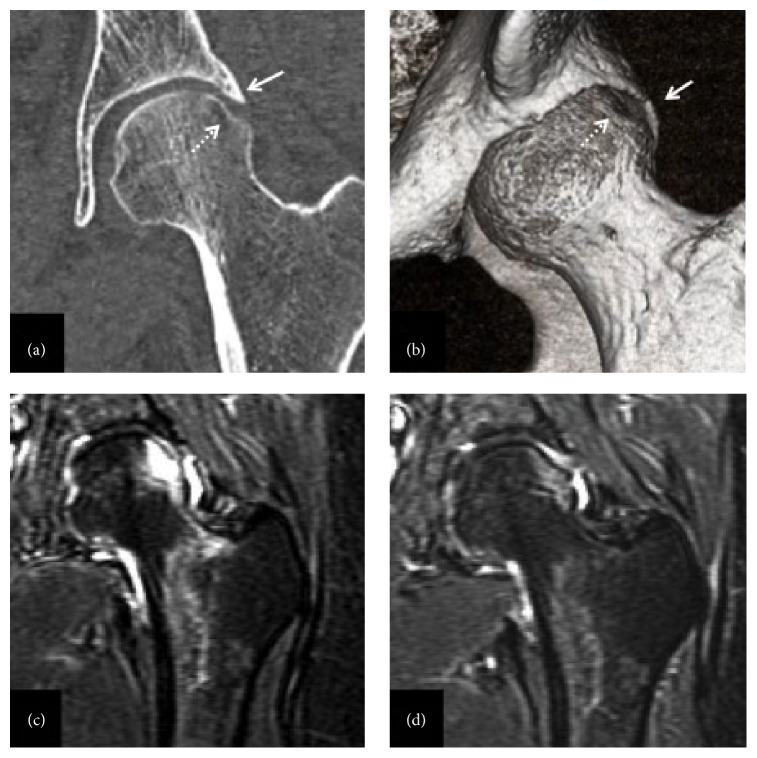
Partial collapse of the femoral head and serial MRI images. (a) Coronal computed tomography (CT) image after 4 weeks of therapy shows subchondral collapse (white dotted arrow) at the antipodes of the lateralized rim (white arrow). (b) A subchondral insufficiency fracture (SIF) is evident at the posterolateral aspect of the femoral head in the oblique three-dimensional CT view. STIR images show that the high intensity lesion (c) is smaller after 10 weeks of therapy and (d) has almost disappeared after 4 months of therapy.
